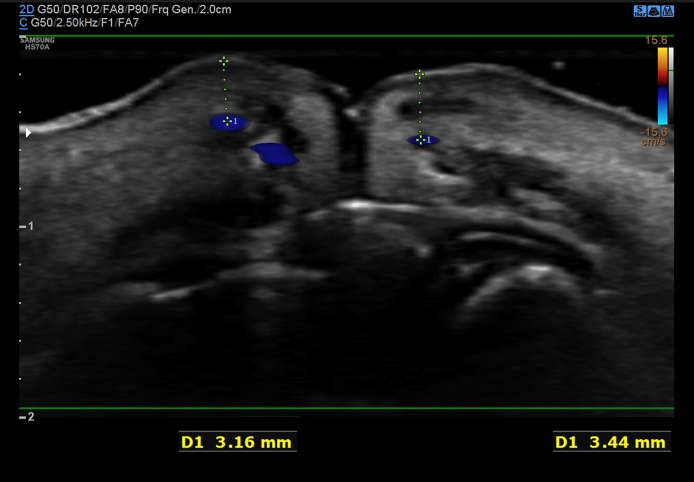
Superior labial artery (SLA) and inferior labial artery (ILA) in sonography before the injection for a 64-year-old female. The sonography probe applied on right apex of Cupid's bow vertically. On the right of image, upper lip was seen and a SLA located under the par marginalis of OOm, 3.16 mm from dry mucosa. Other SLA was found inside of OOm. On the left of image, ILA located 3.44 mm from dry mucosa. The injection layer was submucosa. The injection site was ∼3 mm away from the SLA and ILA.
